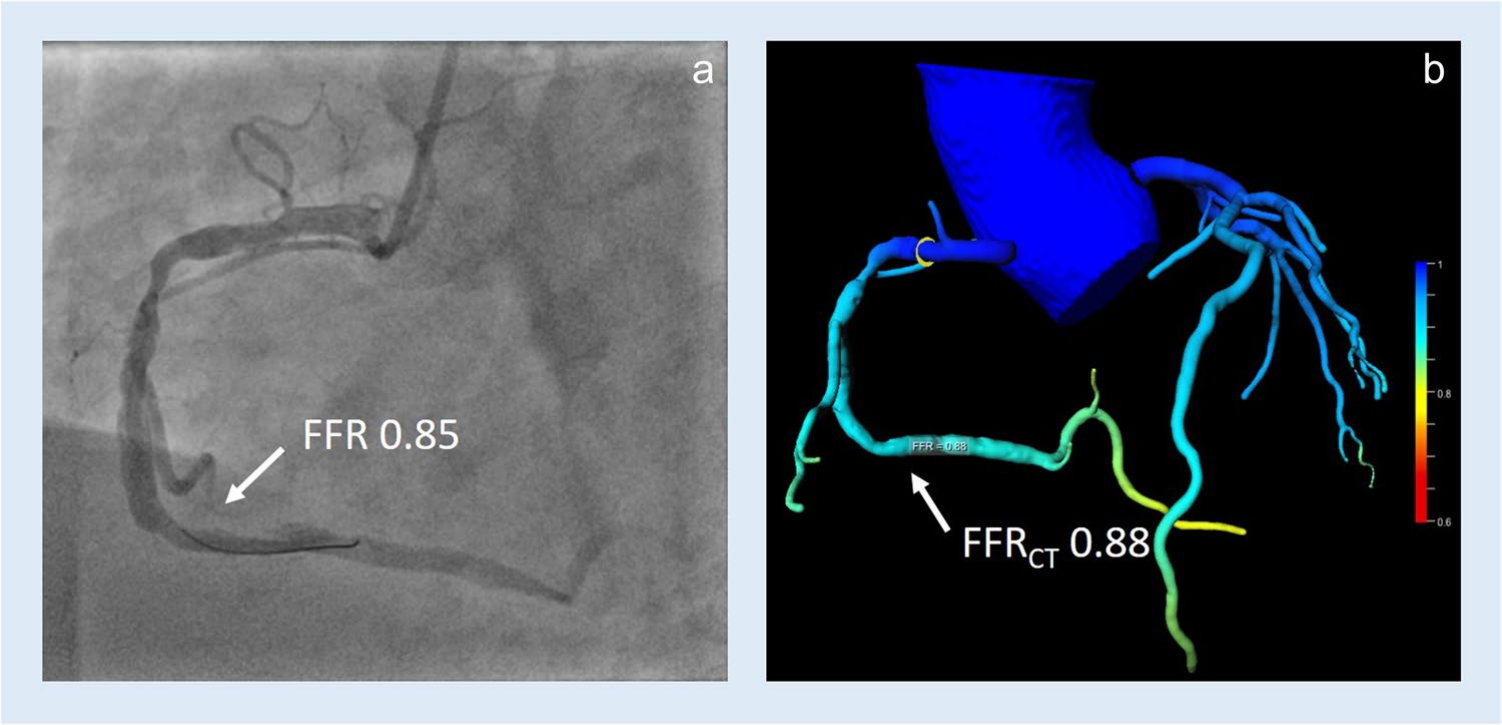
a Invasive angiogram with an FFR wire placed in the distal third of the right coronary artery. b 3D CT reconstruction of the coronary tree showing FFR_CT_
